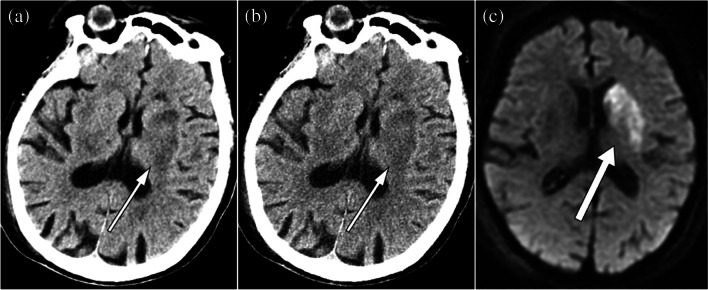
Unenhanced head CT (a, b) of an 85-year-old female patient with acute infarct in the left basal ganglia region (white arrows). The time interval from onset to CT examination was 9 h. The conspicuity of this acute infarct was rated as 3, 3, and 4 in DLR (a) and 2, 3, and 2 in Hybrid IR (b) by readers 1, and 2, and 3 respectively. Window level / width is 25 / 50 Hounsfield unit for both (a) and (b). Diffusion-weighted image is also shown in (c)
